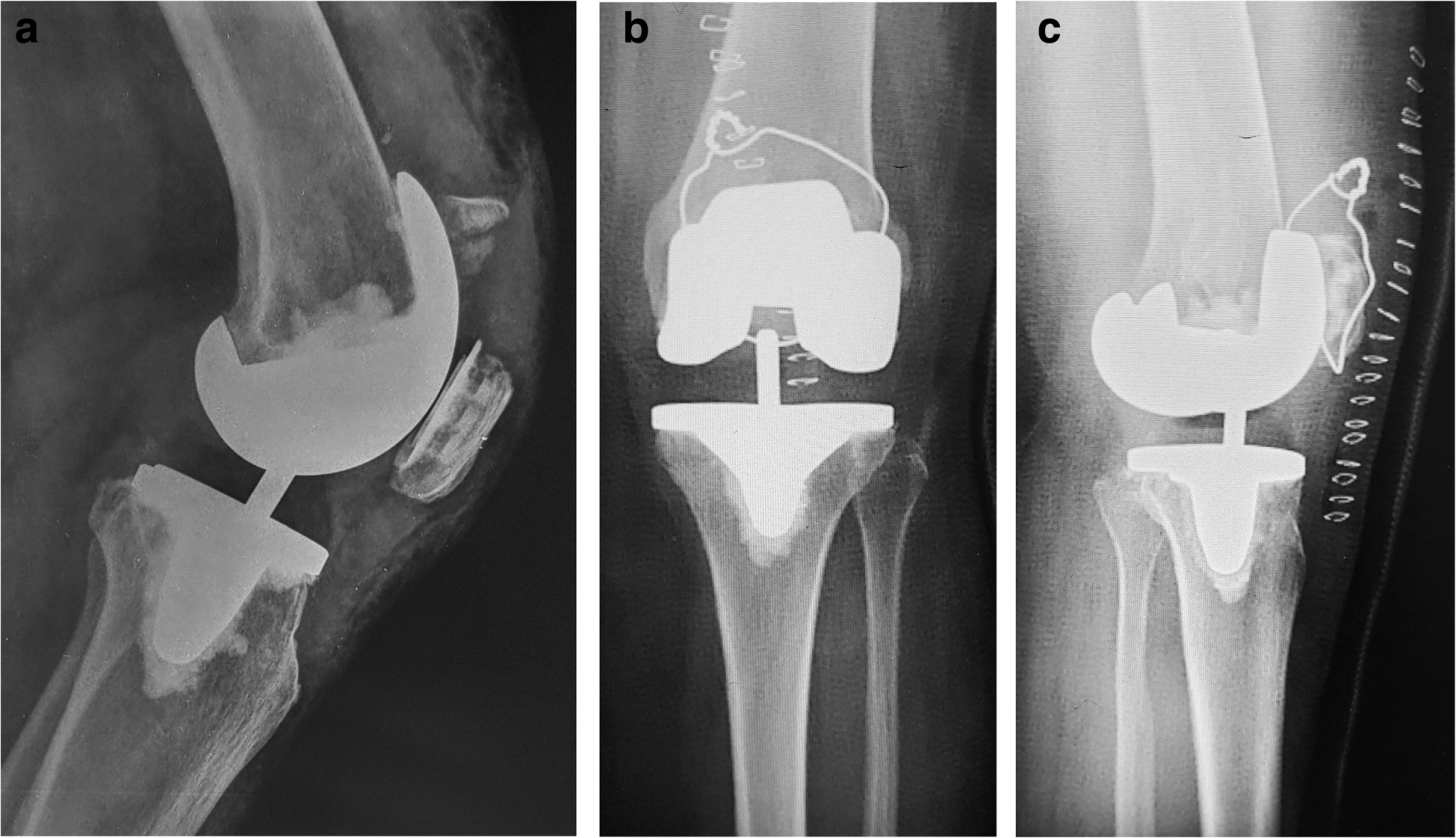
A 68-year-old female patient who suffered a peri-prosthetic patella fracture (PPPF) in her left knee (case 1 in Table 1). a Preoperative lateral X-ray shows a PPPF with extensor mechanism rupture and rotating hinge knee system (Depuy) with stable patellar implant. b Postoperative anteroposterior view shows the fracture is reduced and fixed with cerclage wiring. c Lateral X-ray shows good approximation
